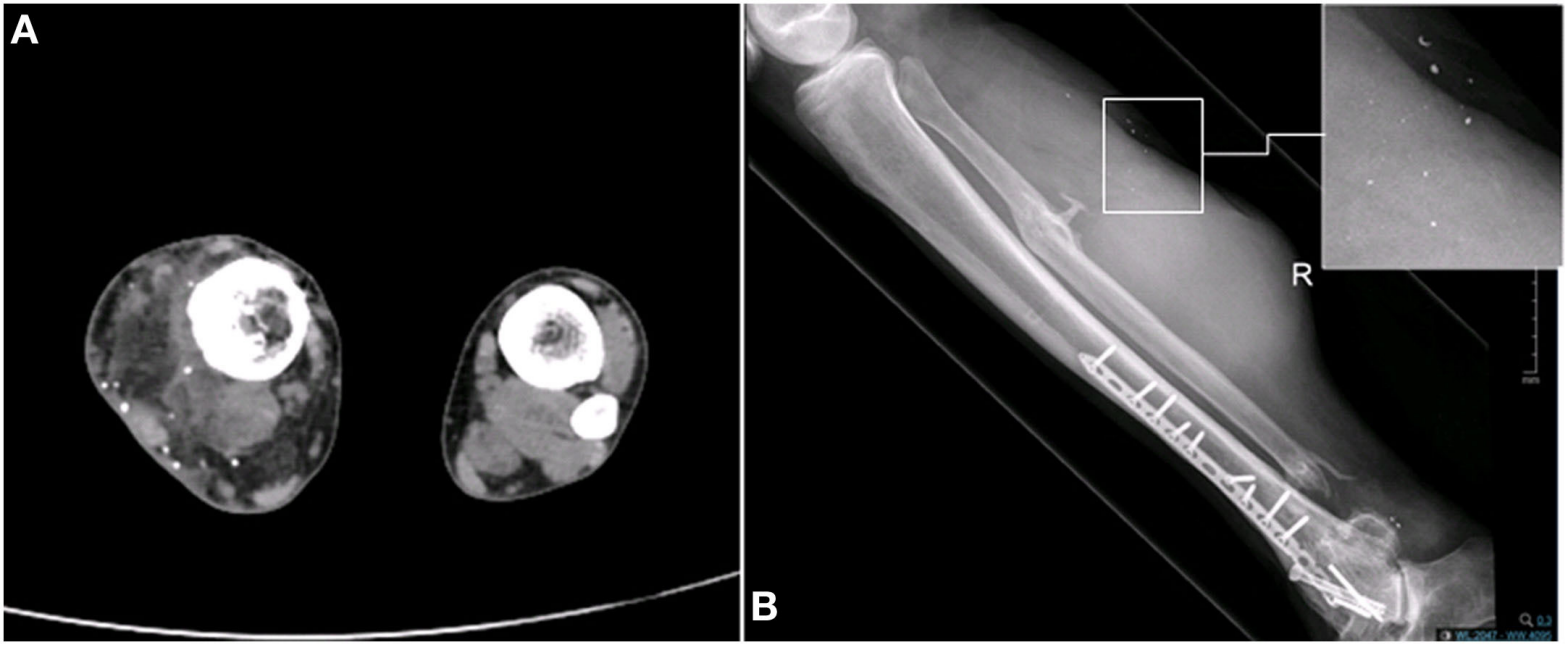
Existence of metal fragments in the area of the sarcoma in the right calf. (A) CT scan. (B) X-ray.
